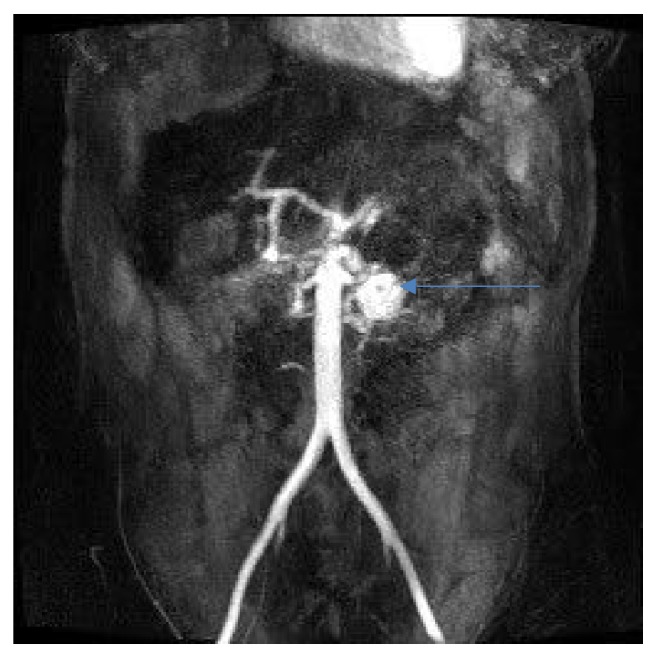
Follow-up MRI angiography showing recanalization of PAAA.
